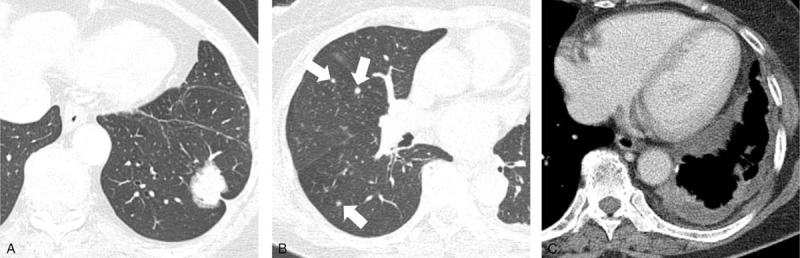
A 67-year-old female patient who underwent left lower lobectomy for pulmonary adenocarcinoma manifesting as a part-solid GGN. A, Preoperative chest CT shows a part-solid GGN in the left lower lobe of the lung. The whole nodule including GGO parts and its solid part were measured as 3.1 and 2.4 cm, respectively. B, C, Follow-up chest CT performed 4 years and 10 months after surgery shows multiple solid nodules in both lungs (arrows) and irregular pleural thickening with effusion.
